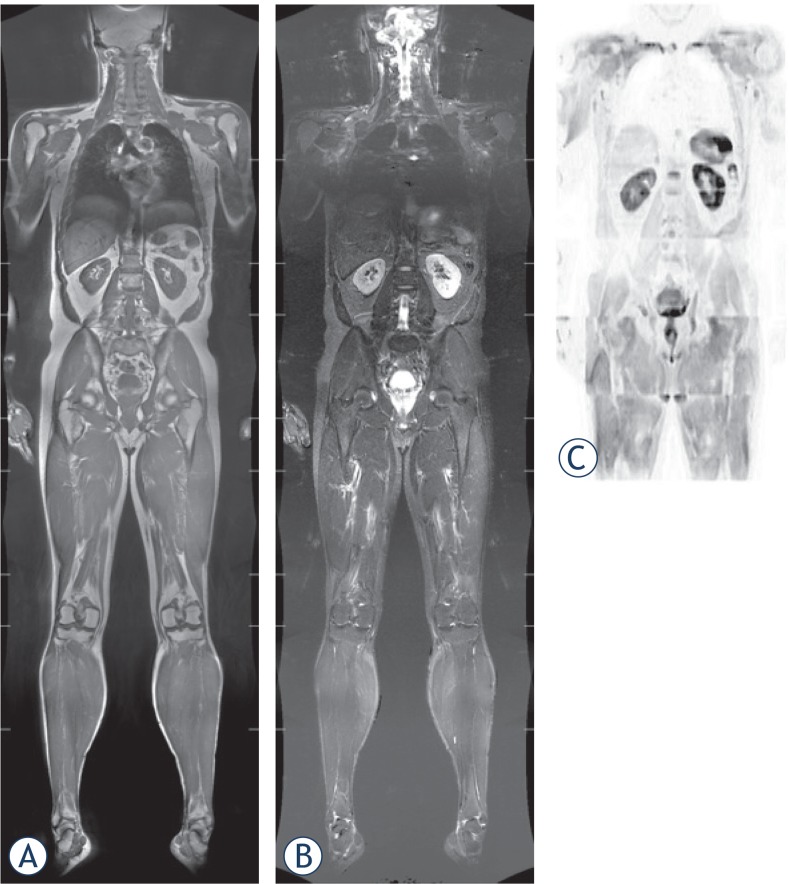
Whole-body MRI acquired on a dedicated whole-body MRI system and matrix coils in asymptomatic, smoker male 46-year-old patient, coronal (A) T1, (B) STIR and (C) DWI images.
